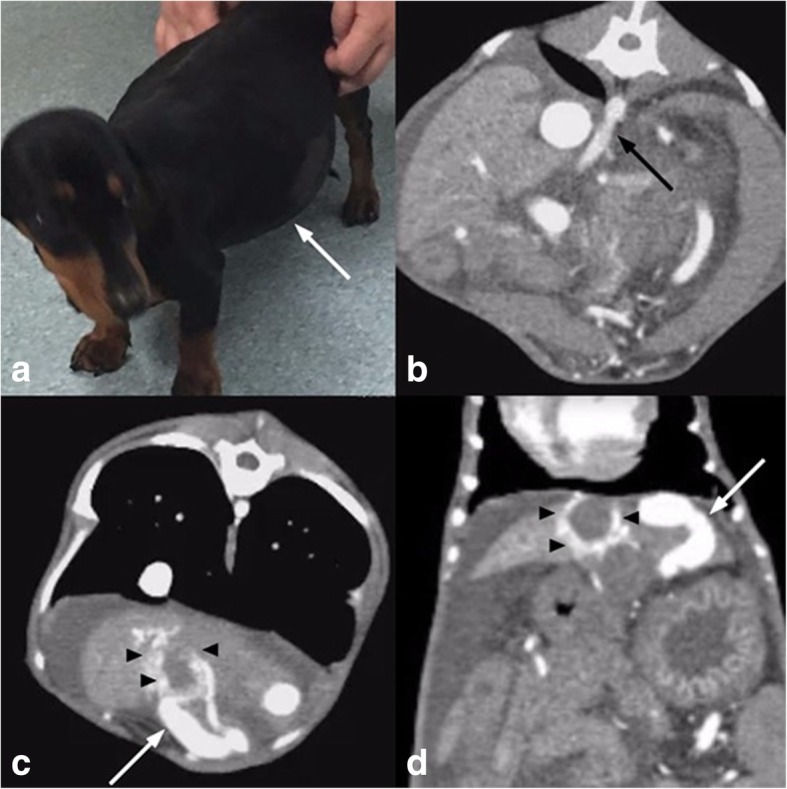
Patient 1 presenting with a) tense ascites (arrow) and biochemical hepatic dysfunction. b) Preoperative CT angiogram demonstrating an enlarged celiac artery (arrow) and extensive ascites. c) and d) demonstrate an AVM nidus surrounding the gallbladder fossa (arrow heads) with an enlarged and arterialised draining portal vein branch (arrow)
